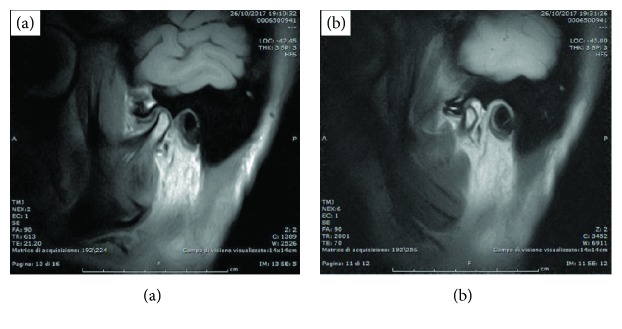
MRI performed in closed (a) and open mouth (b) position during the first visit (T0).
